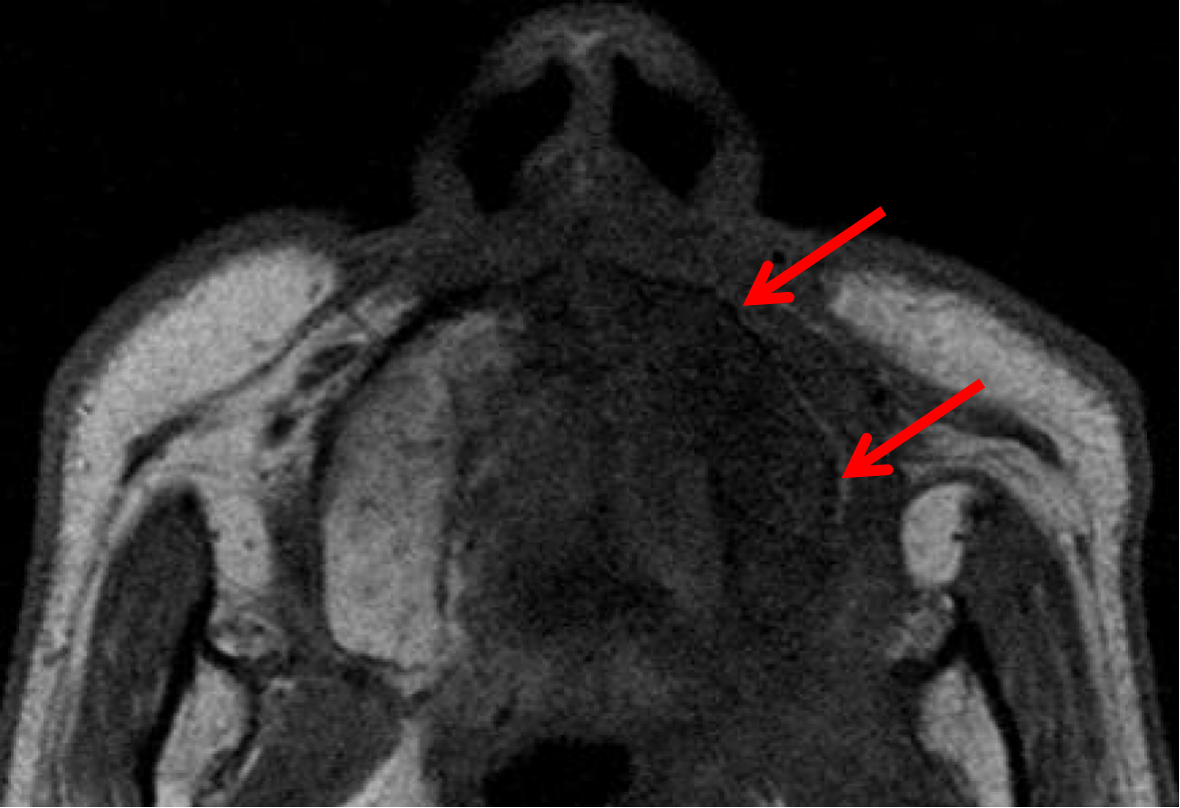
Representative magnetic resonance image (MRI) of the maxillary osteoradionecrosis. T1-weighted axial MRI showed low signal appearance in the left maxilla (red arrows).
